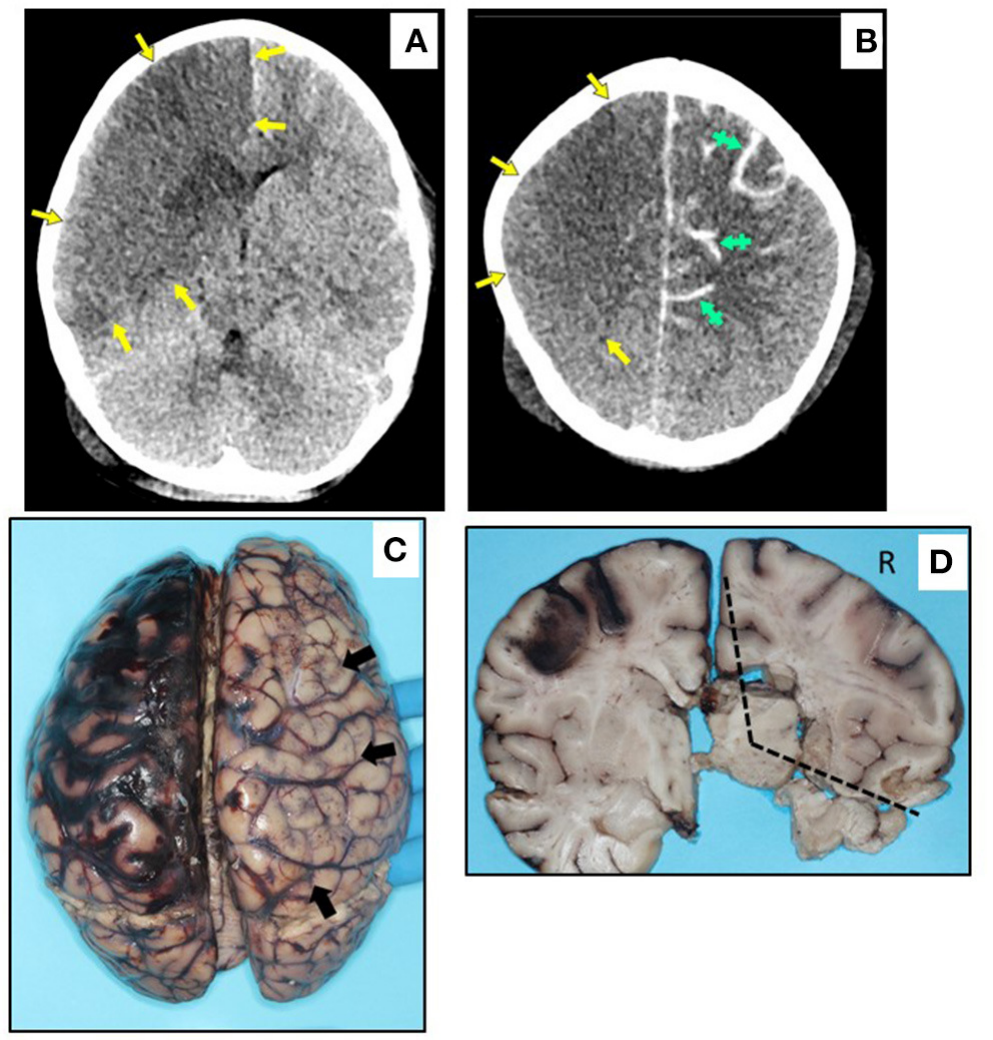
In vivo* CT imaging and macroscopic neuropathology in Case 5. (A,B) Axial non-contrast CT images, displayed in radiological orientation convention. (A) Broad area of hypodensity throughout entire right MCA and ACA distributions (yellow arrows) at level of mid-basal ganglia, reflecting cytotoxic edema in a broad area of infarction; areas of even lower density in caudate head and anterior lenticular nucleus, suggesting older or more established infarcts; minimal midline shift despite infarct volume. (B) Right MCA and ACA infarct at level of upper centrum semiovale, extending more superiorly to the vertex (yellow arrows); additional parenchymal hypodensity on left side, with increased sulcal density (green hatched arrows) reflecting diffuse subarachnoid hemorrhage. (C) Dorsal view of brain, with acute right carotid territory infarct (arrows), as well as subarachnoid hemorrhage over left hemisphere. (D) Coronal section at level of basal ganglia, showing infarct (R = right, dotted lines), and hypoperfusion of left watershed zone with reperfusion hemorrhage.
